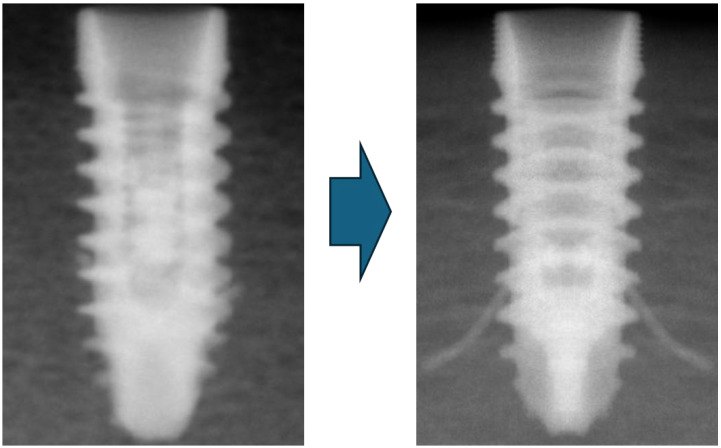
X-ray picture of the dental implant inserted before and after the barrettes were deployed.
